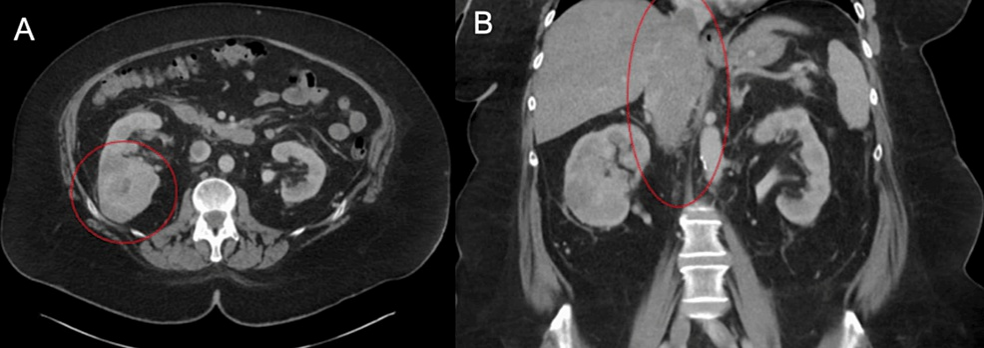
CT imaging of the abdomen and pelvis at initial presentation. (A) Axial view with 9 cm right renal mass. (B) Coronal view showing superior extent of occlusive thrombus above the diaphragm.
